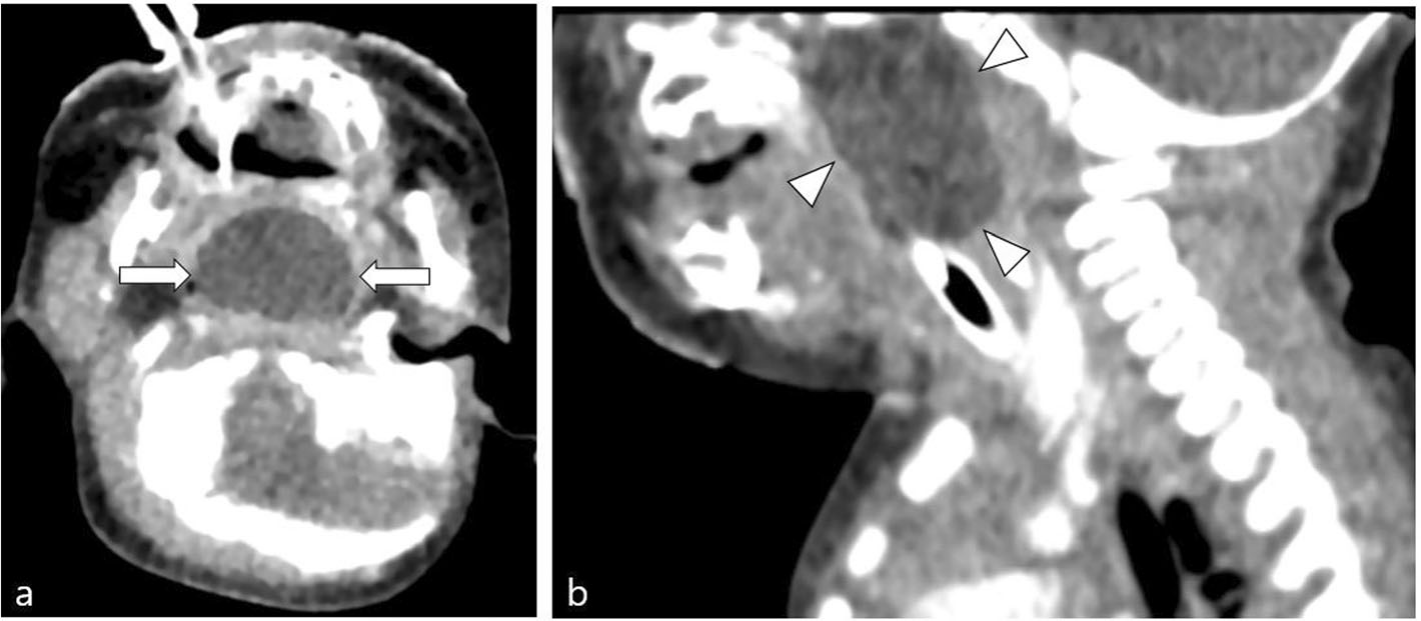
Contrast-enhanced computed tomography (CT) scan of the airway. a Axial CT scan obtained at the level of the oropharynx demonstrates a large cystic lesion (2 × 2.8 cm) causing airway obstruction (arrows). b Sagittal reconstructed image shows a midline cystic lesion involving the choana, nasopharynx, and oropharynx (arrowheads)
